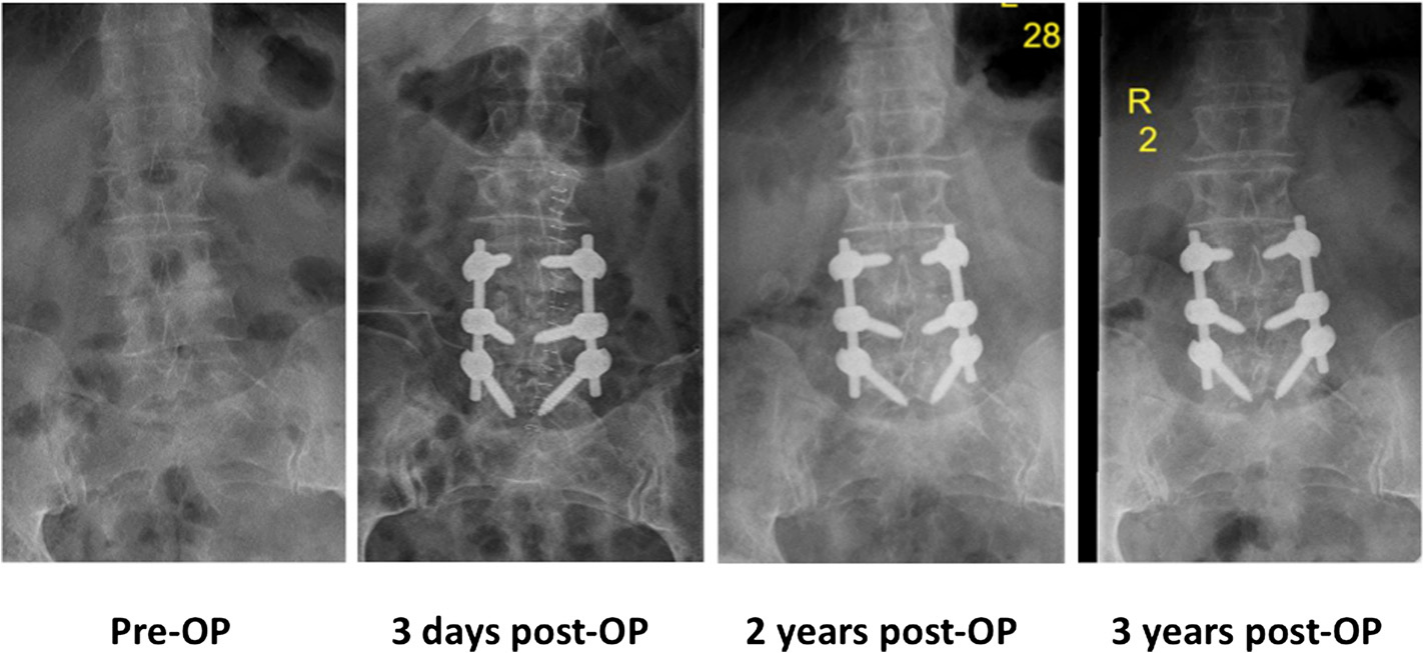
Pre- and post-operative conventional X-rays in the a.p. view (*upright standing*) throughout the 3 year follow-up
